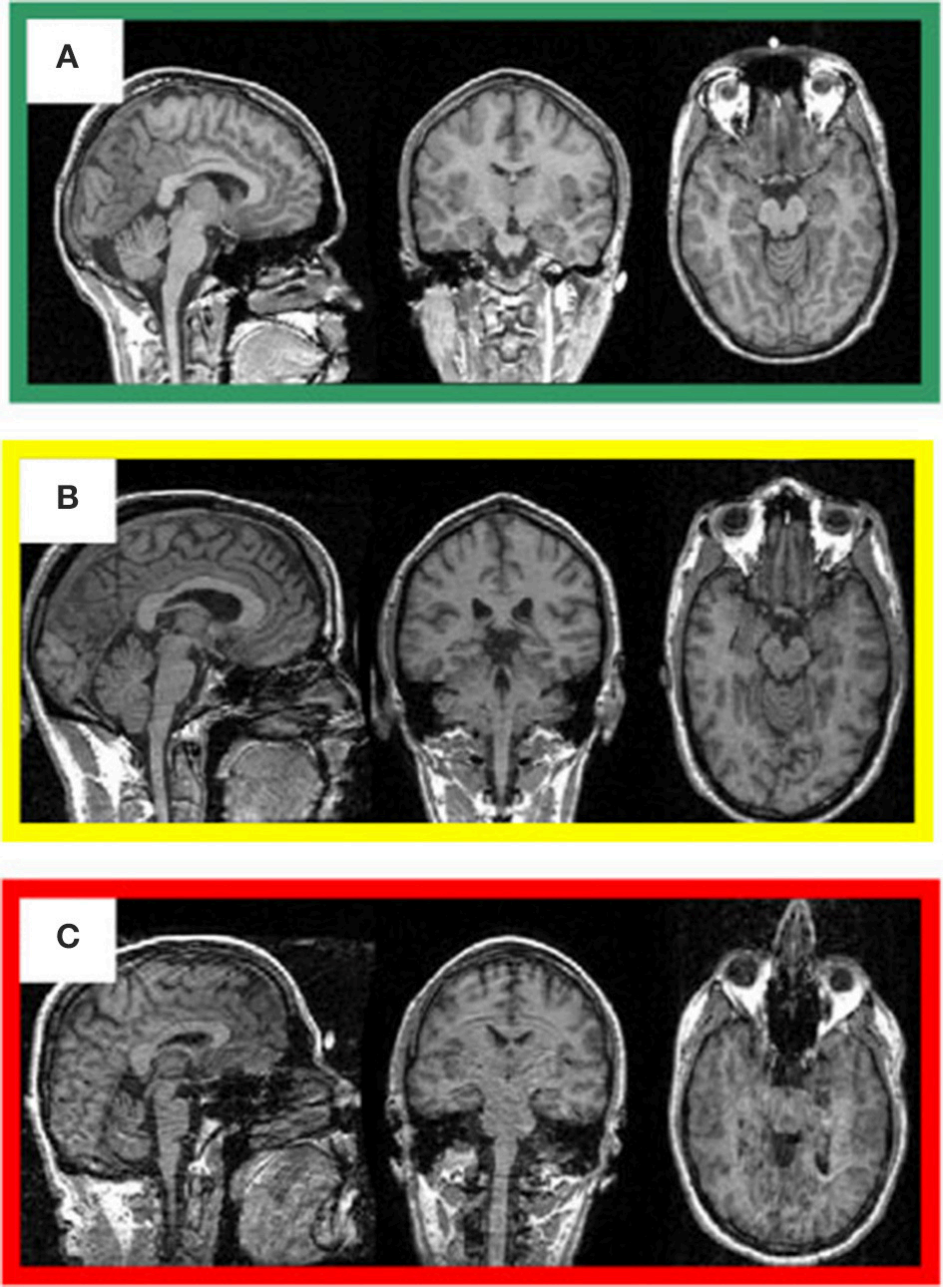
Representative 3D-MRI volumes are presented with corresponding image quality. (A) Green indicates usable and has excellent contrast between gray and white matter. (B) Volume contains slight ringing, was labeled yellow at stage I and usable at stage II. (C) Red indicates not-usable, has ringing, eye, and head movement.
